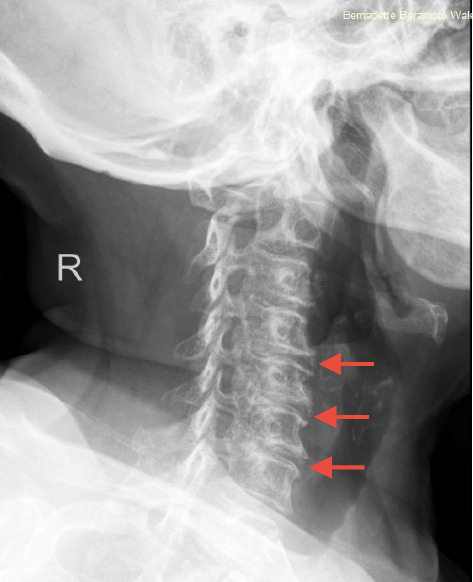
A cervical spine X-ray of the patient reveals moderate-to-severe multilevel degenerative changes in the C4-C5, C5-C6, and C6-C7 intervertebral regions (arrows).
